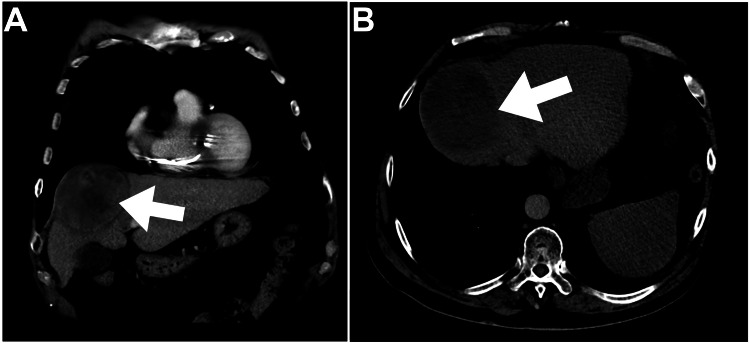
Baseline abdominal CT Coronal, and axial hepatic views of abdominal CT depicted a large 7.5 cm segment VIII hepatic mass (arrows).
